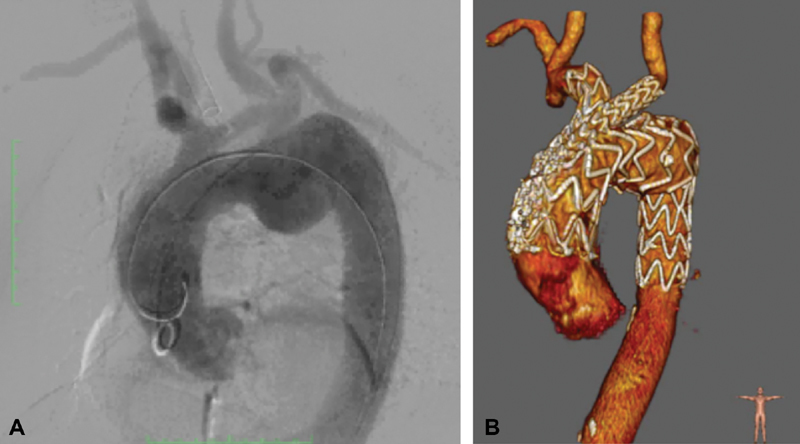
Angiography (panel B) and computed tomography scan—three-dimensional view (panel A) pictures following the procedure.
